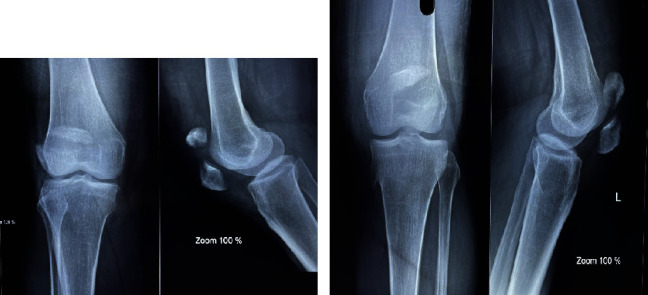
(a) X-ray of the right knee. (b) X-ray of the left knee with incidental osteochondroma.
